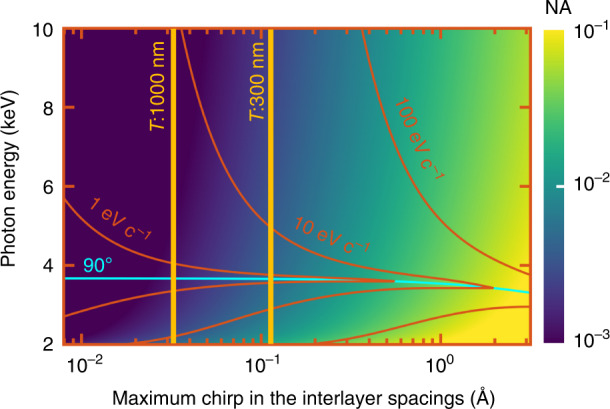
Limits to the obtainable X-ray numerical aperture (NA) due to wave optics and quantum mechanical effects. From wave optics: the focal depth and length are inversely proportional to NA^2^ and NA, respectively. Therefore, there is a lower bound for the NA, because the focal depth should be smaller than the focal length. The two vertical yellow lines delineate the condition of the ratio focal depth⁄focal length = 1 for samples of thicknesses *T* = 1000 and 300 nm. The ratio decreases for larger sample thicknesses and larger chirp in the interlayer spacings. A smaller ratio corresponds to a shorter axial focal region (i.e., a less elongated hotspot). From quantum mechanical considerations: the photon coherence at the focal spot is tied to the electron coherence. The red curves indicate the lower bound for the electron transverse momentum range required for photons to interfere coherently at the focal spot. Generally, a larger electron transverse coherence is needed for achieving a bigger NA. Intriguingly, when the focused X-ray beam is emitted along directions normal to the electron velocity (cyan curve), it requires relatively smaller electron coherence. The calculation is based on the platform of Fig. 2b, with a minimum interlayer spacing of 12.70 Å
